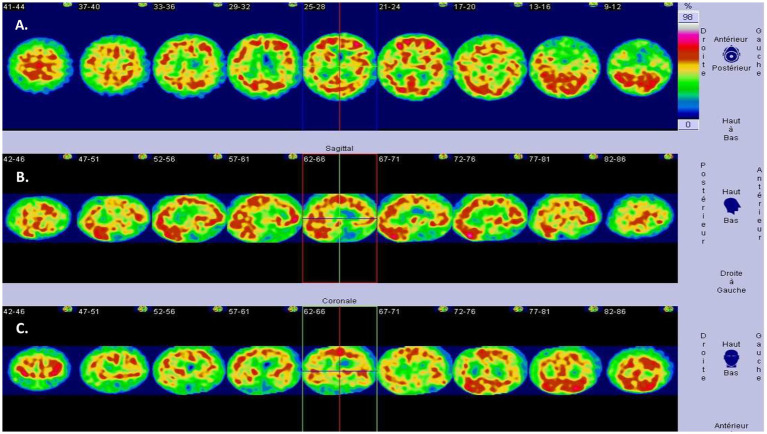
SPECT HMPAO (99mTc) study of regional blood flow. Highly heterogeneous distribution of the cerebral cortex with biparietal and bitemporal hypoperfusion [(A) Axial, (B) Sagittal, (C) Coronal] extending to the posterior frontal regions (low activity in the basal ganglia is also noted).
